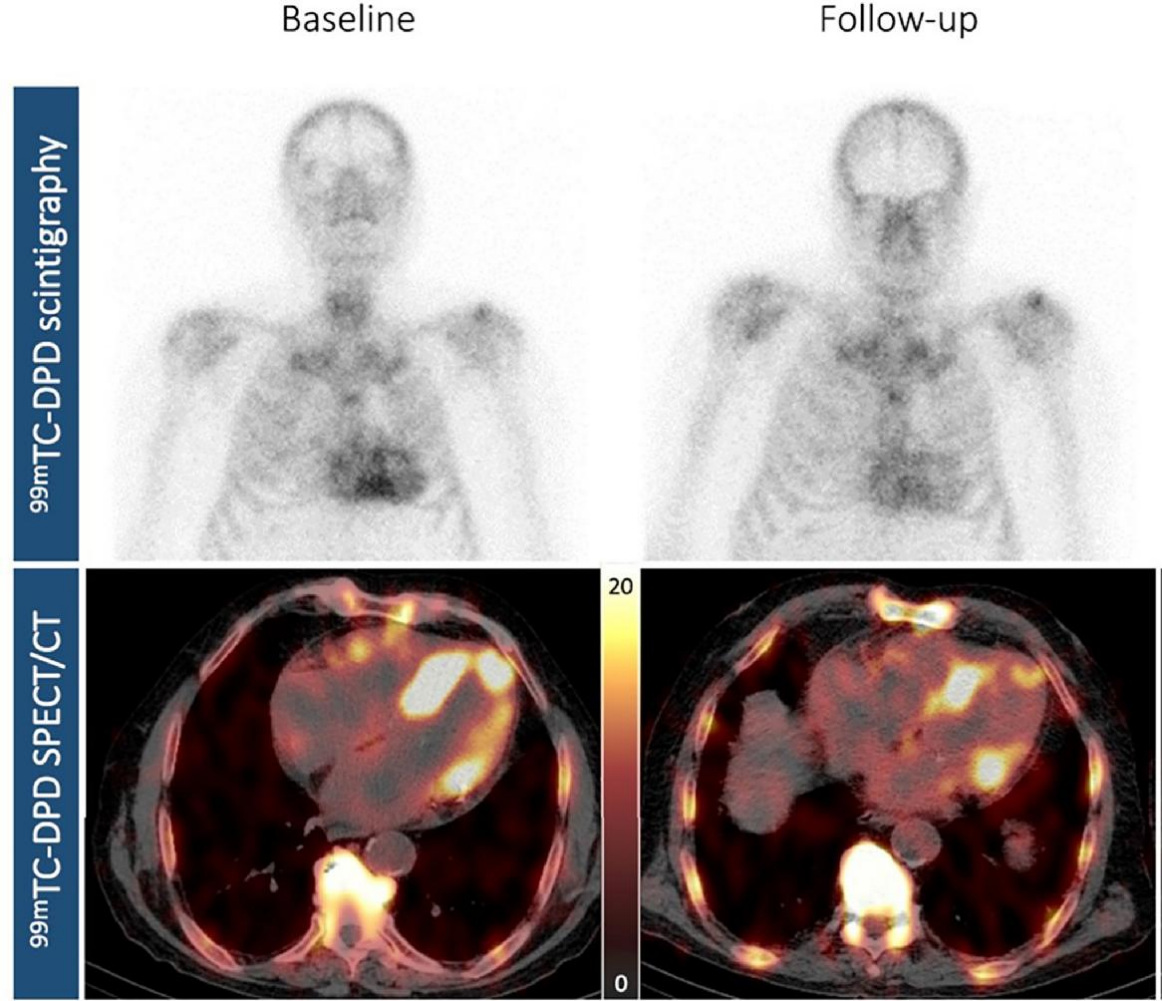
Regression of wild-type ATTR amyloidosis under tafamidis therapy. Visualization of response to tafamidis treatment (61 mg once daily) by nuclear imaging. Planar whole-body DPD-scintigraphy in the upper panels and axial ^99m^Tc-DPD-SPECT in the lower panels, with baseline findings on the left (upper left panel, Perugini grade 3, lower left panel, clear tracer uptake in the left ventricular walls; peak cardiac standardized uptake value: 12.40 g/mL) and follow-up findings after approximately 9 months on the right (upper right panel, Perugini grade 2, lower right panel, decreased myocardial uptake, peak cardiac standardized uptake value: 8.56 g/mL). Reproduced from.^22^
